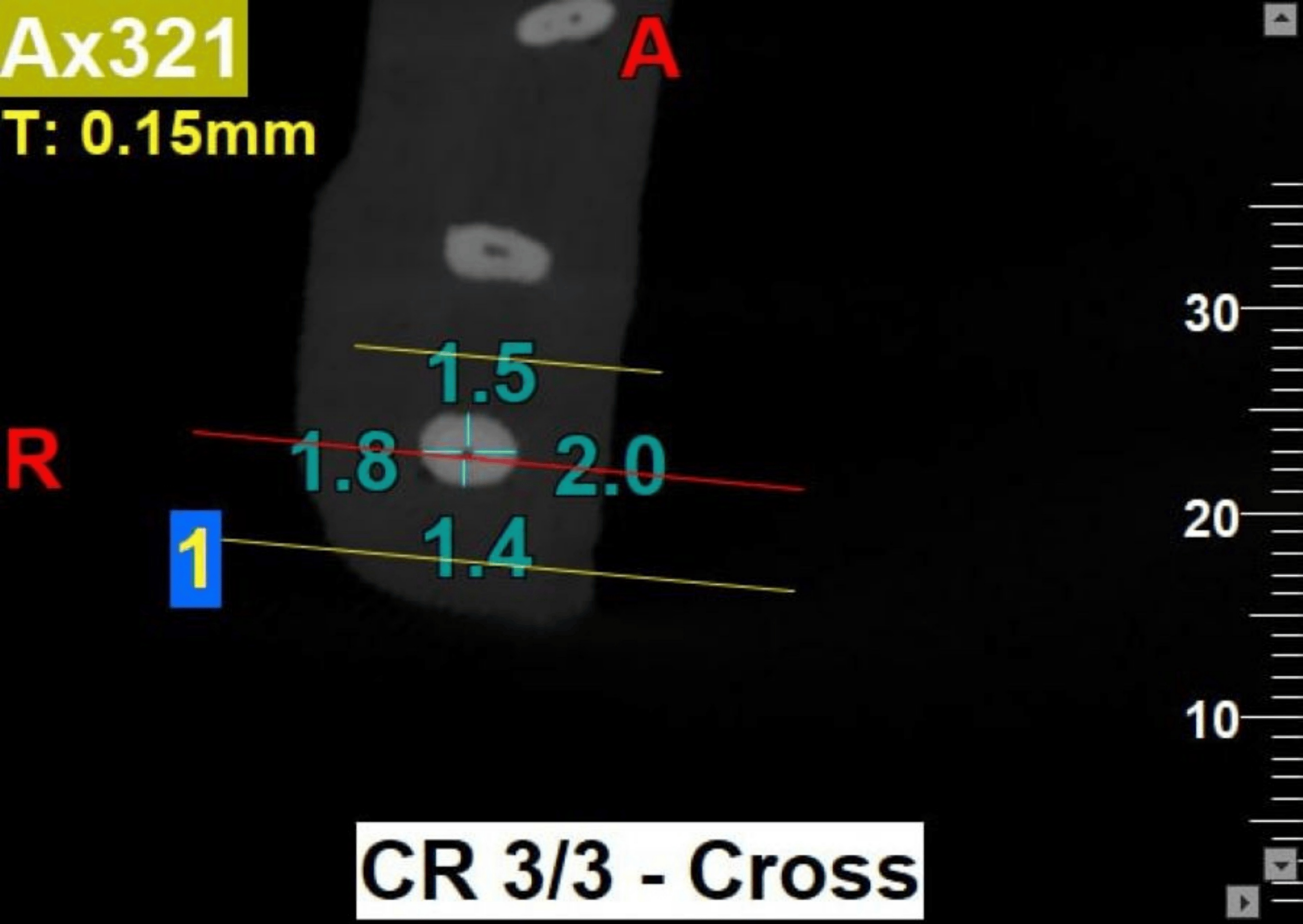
Pre-instrumentation CBCT at 3 mm from pre-determined working length. CBCT: Cone beam computed tomography
